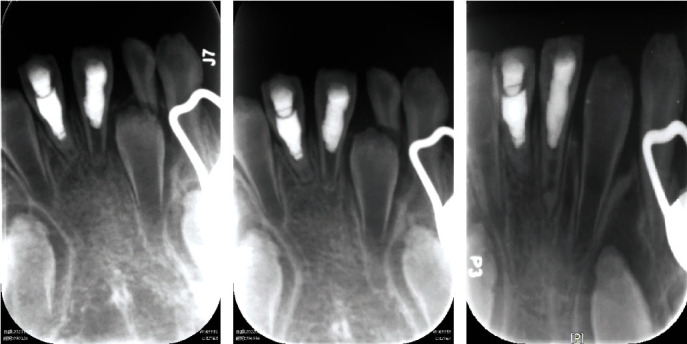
Recall radiographs of the case. (a) One-month postoperative radiograph showed alleviation of periapical radiolucency. (b) Three-month postoperative radiograph demonstrated healing of periapical lesion. Maturation of root was not evident. (c) Twelve-month postoperative recall radiograph clearly showed elongation of root and thickening of dentinal canal walls, especially when compared with tooth 41, which was treated with apexification. Dentinal bridge and closure of root apex were also obvious.
